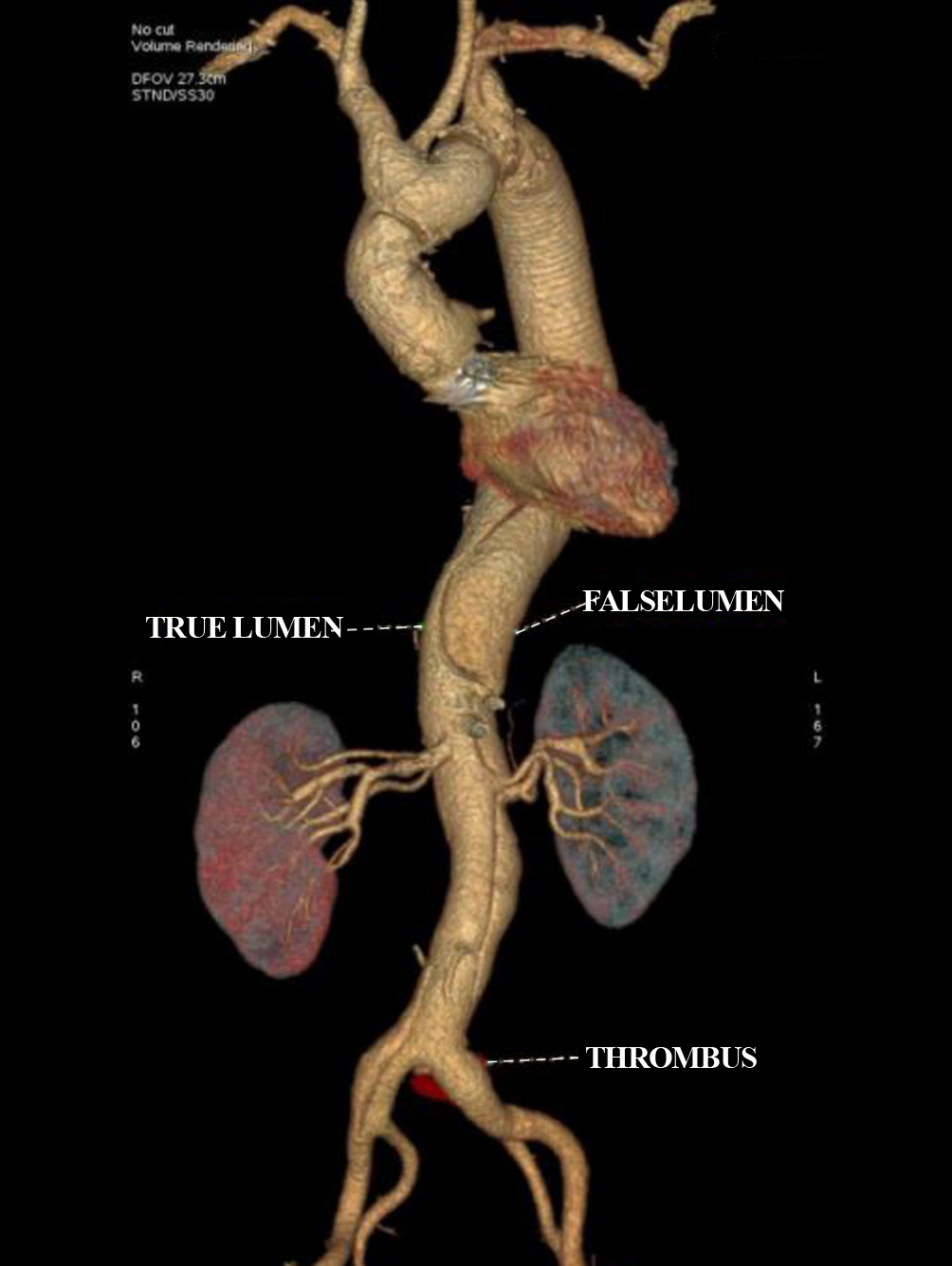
Postoperative CT scan showing a complete replacement of ascending, arch and proximal descending thoracic aorta.
